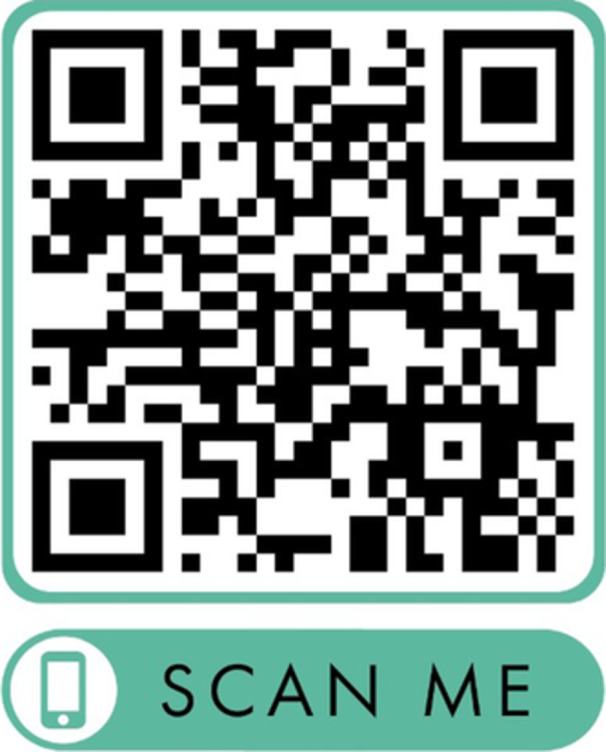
### Radiographic Evaluation
